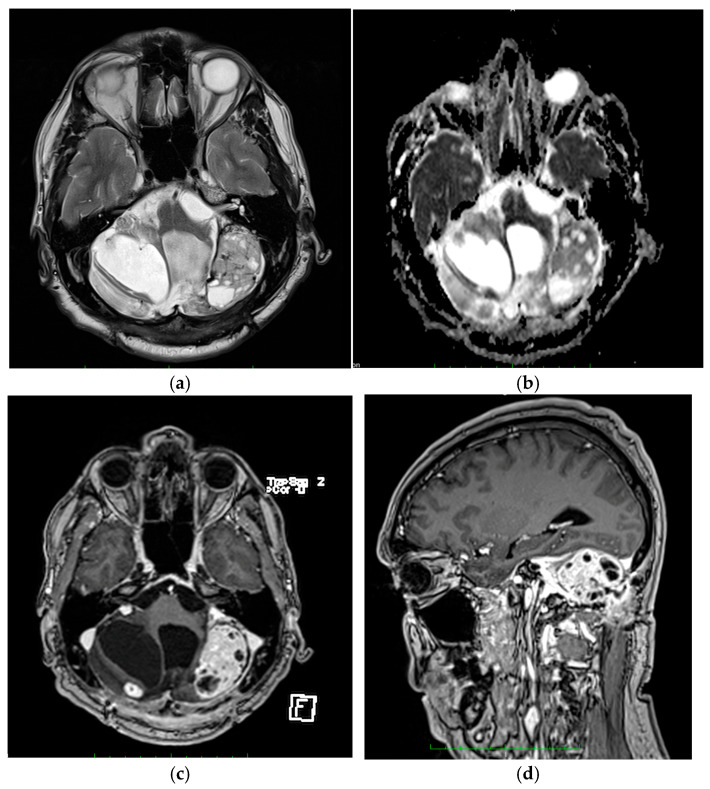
MRI results of patient one before surgery. (a) Image of an axial section though the T2 sequence, revealing a tumor process, which completely occupies the left cerebellar hemisphere, isointense, heterogeneous and with cystic areas inside. (b) Image of an axial section, diffusion sequence, which reveals the tumor process with marked restriction. (c) Image of an axial section, postcontrast T1 sequence, which highlights the tumor process located at the level of the left cerebellar hemisphere with intense and heterogeneous gadolinium contrast uptake; two more gadolinophilic nodules are present in the right pontocerebellar angle and right cerebellar convexity. (d) Image of a sagittal section, postcontrast T1 sequence.
